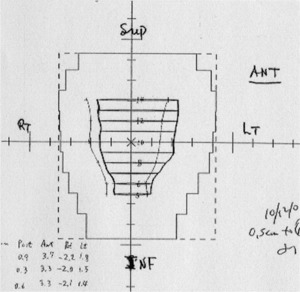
Mapping of prostate contours on treatment day onto planning AP beam's eye view. The solid outline represents the original prostate position (CTV) as in planning CT. The thin outline represents the new prostate position on treatment day relative to (uncorrected) MLC aperture.
